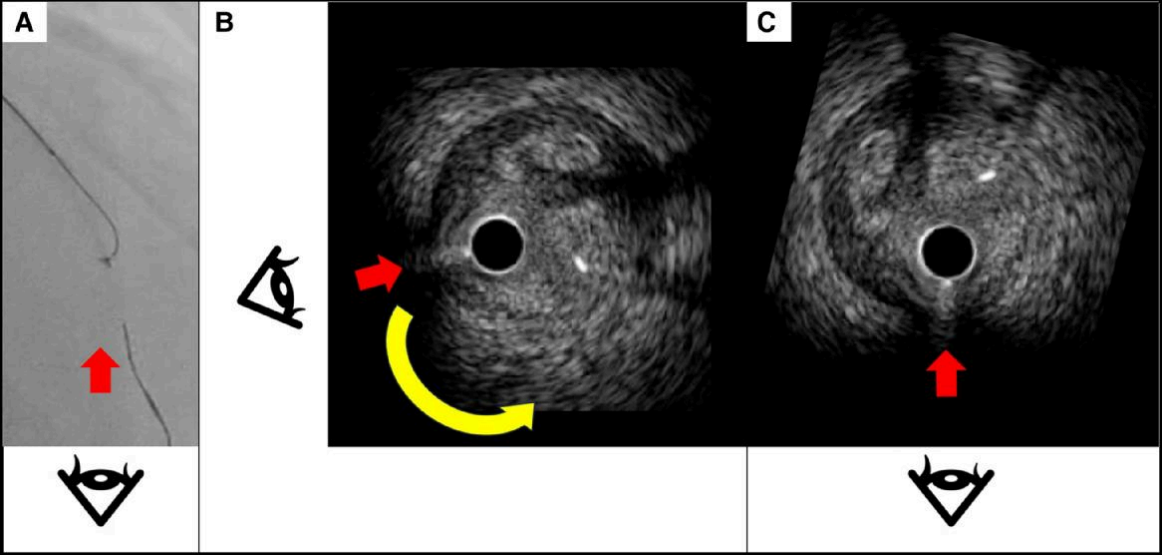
Integrating fluoroscopic and IVUS images to torque the guidewire in a same direction. (*A*) The fluoroscopic viewing direction is defined as 6 o’clock in mid-left anterior descending coronary lesions from the cranial fluoroscopic view. (*B* and *C*) To align the viewing direction on fluoroscopy and intravascular ultrasound (IVUS), IVUS images are rotated 70° counterclockwise. Thus, the downward direction on the fluoroscopic image corresponds to the direction from 2 to 10 o’clock on the IVUS image.
